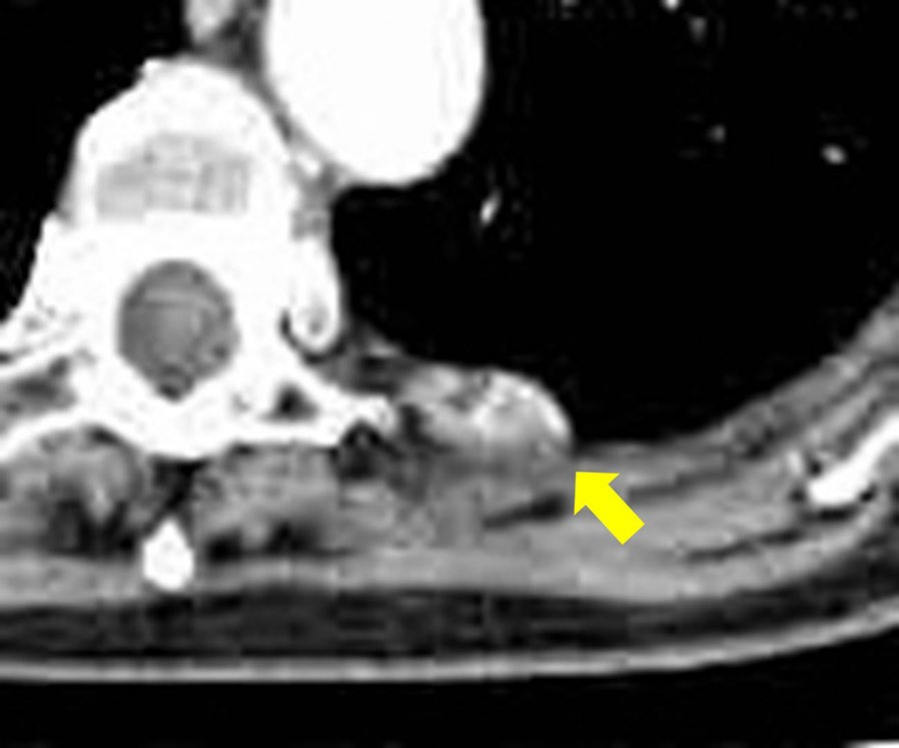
Enhanced chest CT scan findings. Enhanced chest CT showed an about 22-mm-sized heterogeneous enhanced nodule in the left chest wall at the fifth–sixth intercostal level
